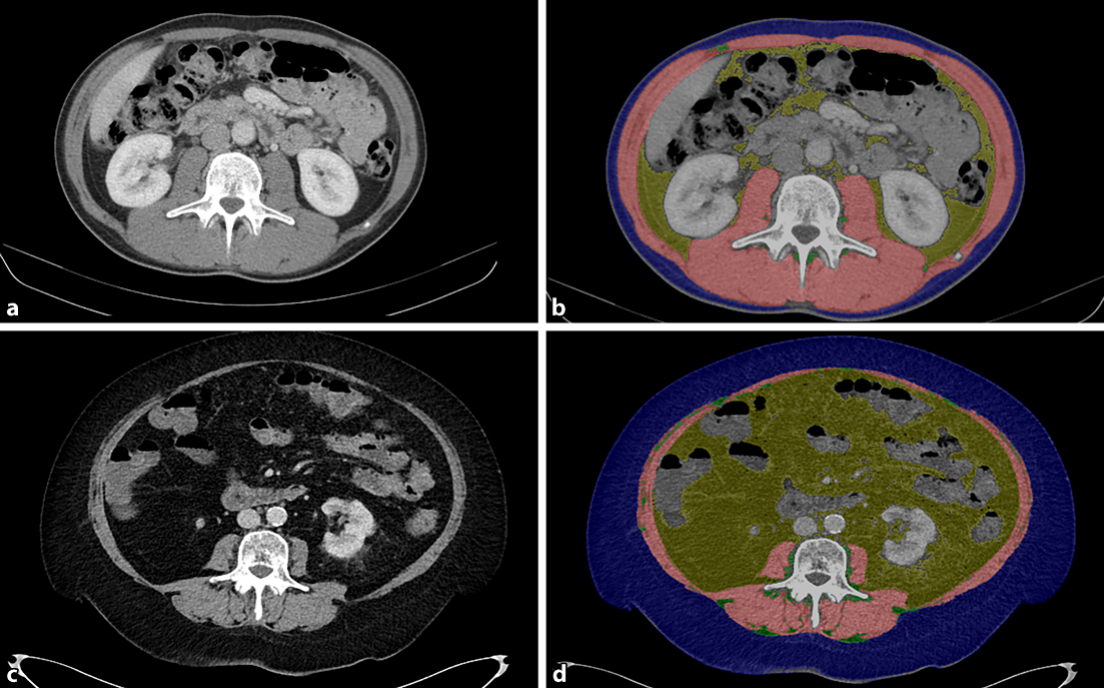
Darstellung der CT-graphisch vermessenen Körperzusammensetzung, Muskelfläche (*rot*), Fläche des viszeralen Fettgewebes (*gelb*), Fläche des subkutanen Fettgewebes (*blau*), Fläche des intramuskulären Fettgewebes (*dunkelgrün*). Patient mit normaler Muskelmasse und geringem viszeralem Fett (a), segmentierte Areale der Körperzusammensetzung (b), Patient mit reduzierter Muskelmasse und deutlicher viszeraler Adipositas (c), segmentierte Areale der Körperzusammensetzung (d)
